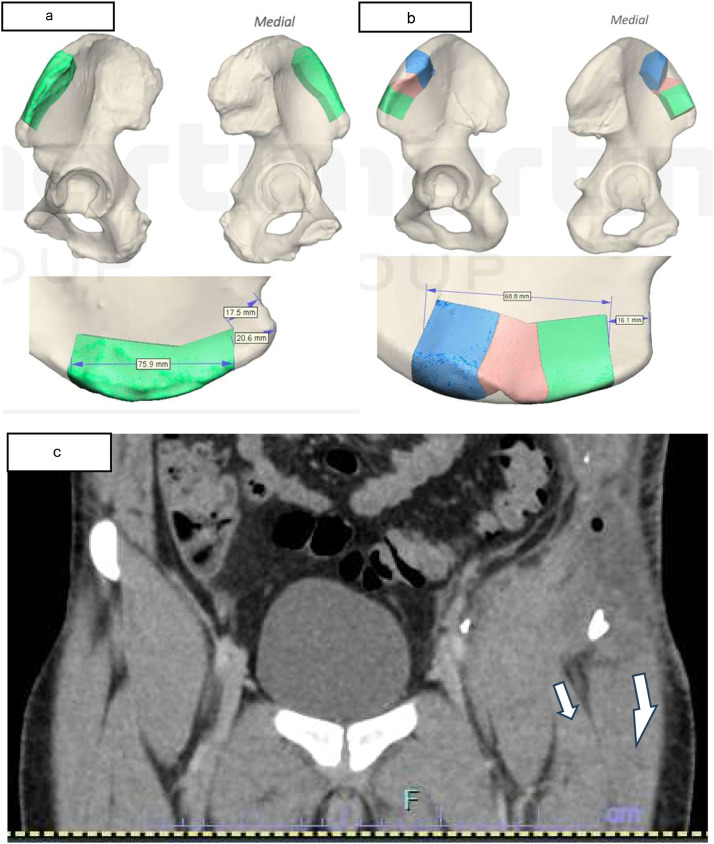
Virtual surgical planning of case 1. b Virtual surgical planning of case 2. c. CT Images showing Sartorius muscle and Tensor fascial latae on white arrows showing a downward traction force.
